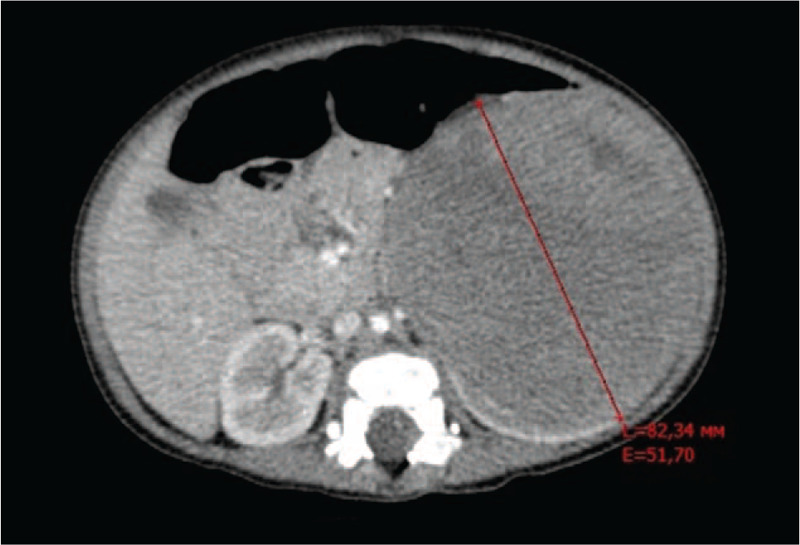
Contrast-enhanced CT scan obtained at the patient's age of 6 months. A large, solid, expansive tumor replaced the left kidney. There was no enlargement of lymph nodes, and the right kidney showed no tumor signs.
